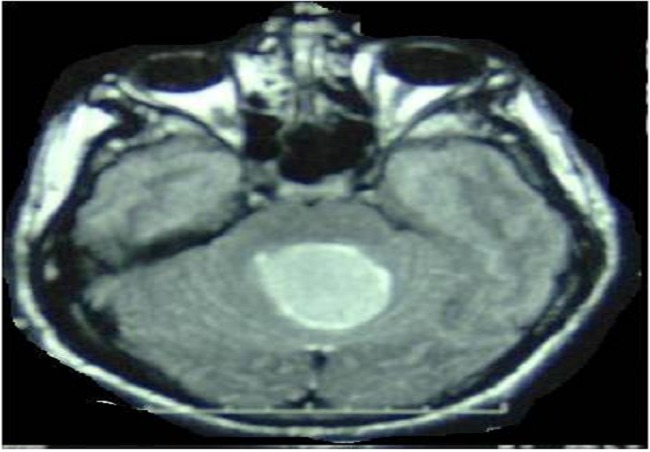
Brain MRI FLAIR, axial sequence, showing a hyperintense signal in the cyst different to CSF signal
